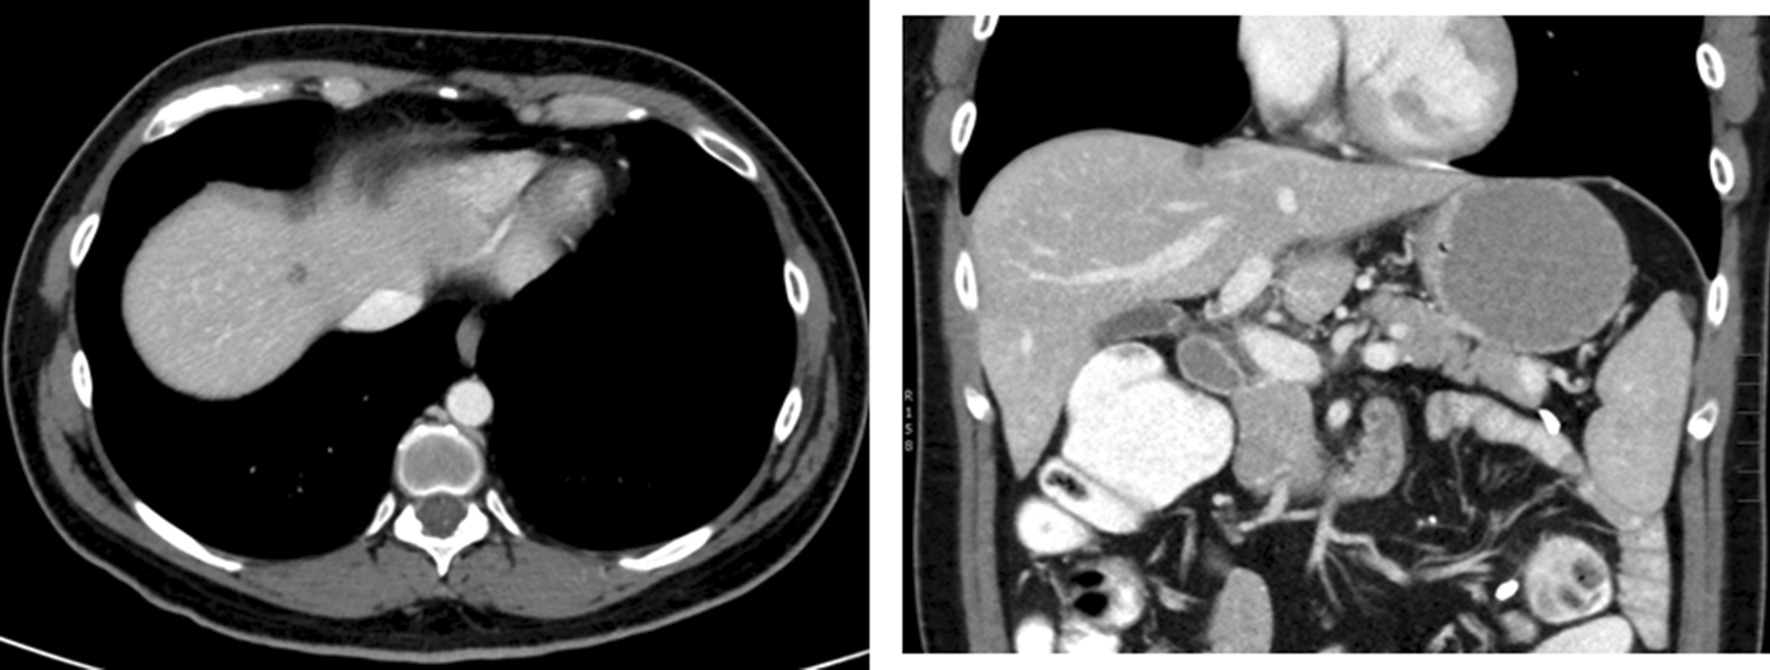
CT of the abdomen showing segment 4A/8 liver lesion
